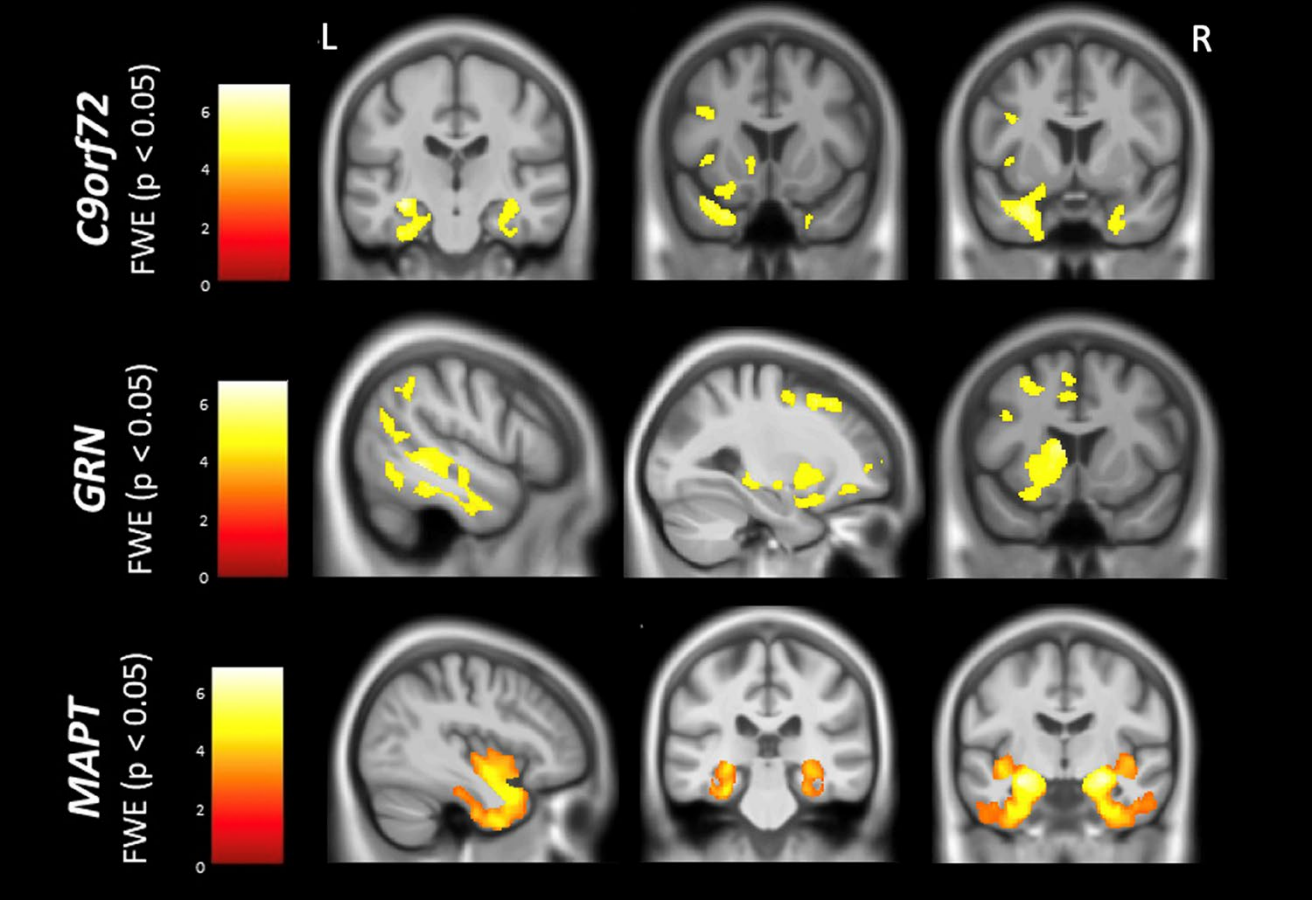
Neural correlates of naming in C9orf72, MAPT and GRN mutation carriers. Results are shown on a study-specific T1-weighted MRI template in MNI space and at *p* < 0.05 for Family-Wise error. Colour bars represent T-values
